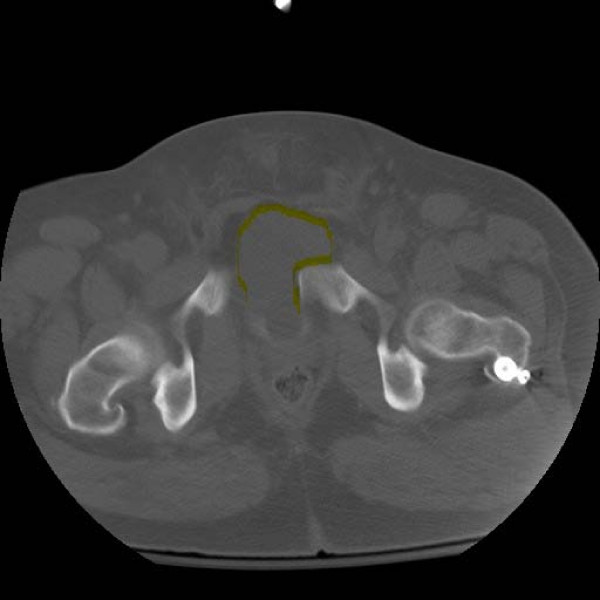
Postoperative CT cystogram showing incomplete reduction of the symphysis with persistence of the bladder incarceration within the pubic symphysis. The herniated bladder has been outlined for clarity.
